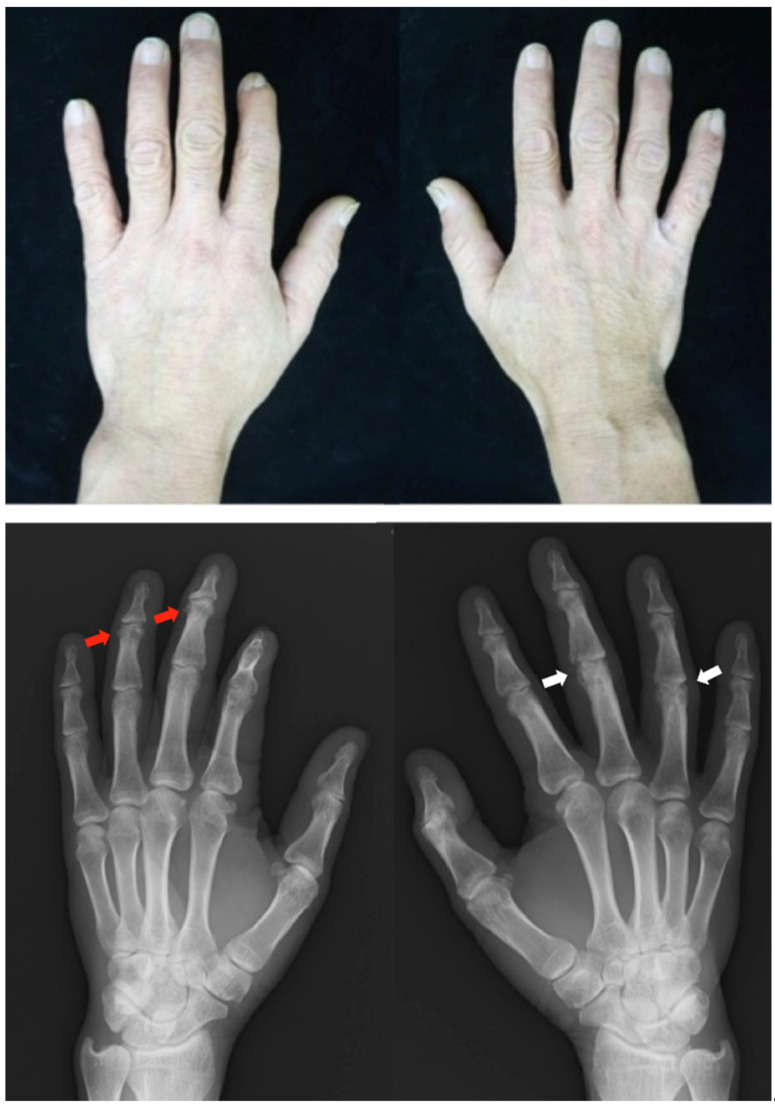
Clinical photographs and radiographs of the hands in case 7. Radiographs show erosions adjacent to periarticular bone proliferation at the collateral ligament and capsular joint attachment site (red arrow). Bone erosions are noted in the right third and fourth PIP joints (white arrow). PIP, peripheral interphalangeal.
